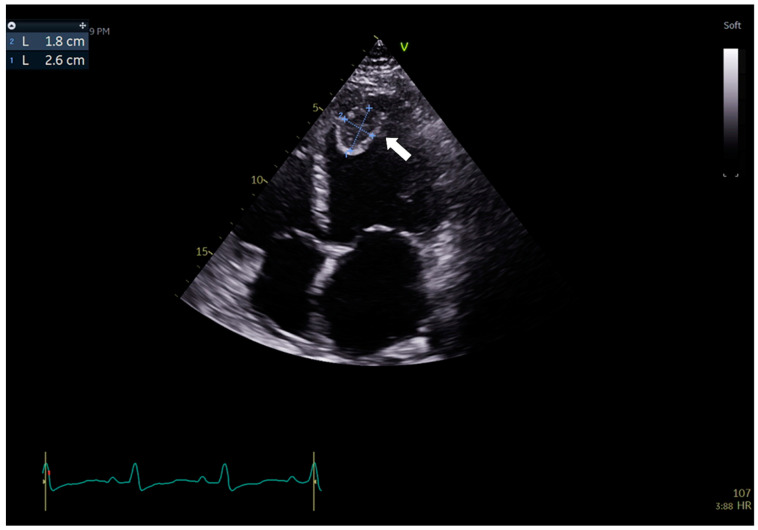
Transthoracic echocardiography (TTE) findings in Patient 3 on admission. There is a severe enlargement of the left heart chambers. The systolic function of LV is profoundly impaired, with the left ventricular ejection fraction (LVEF) calculated at 20%. In the apex of the LV, there is a soft, mobile thrombus with dimensions of 35 × 23 mm (arrow).
